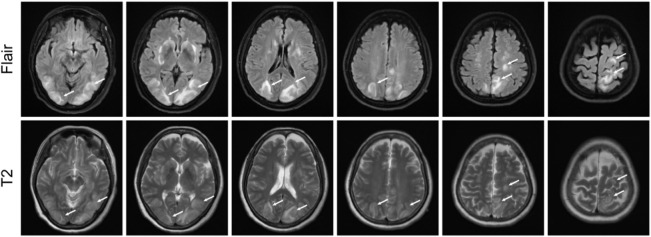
Magnetic resonance imaging results showing possible posterior reversible encephalopathy syndrome. T2 and fluid-attenuated inversion recovery images showing strong signals in bilateral frontal lobes, occipital lobes, and basal ganglia.
